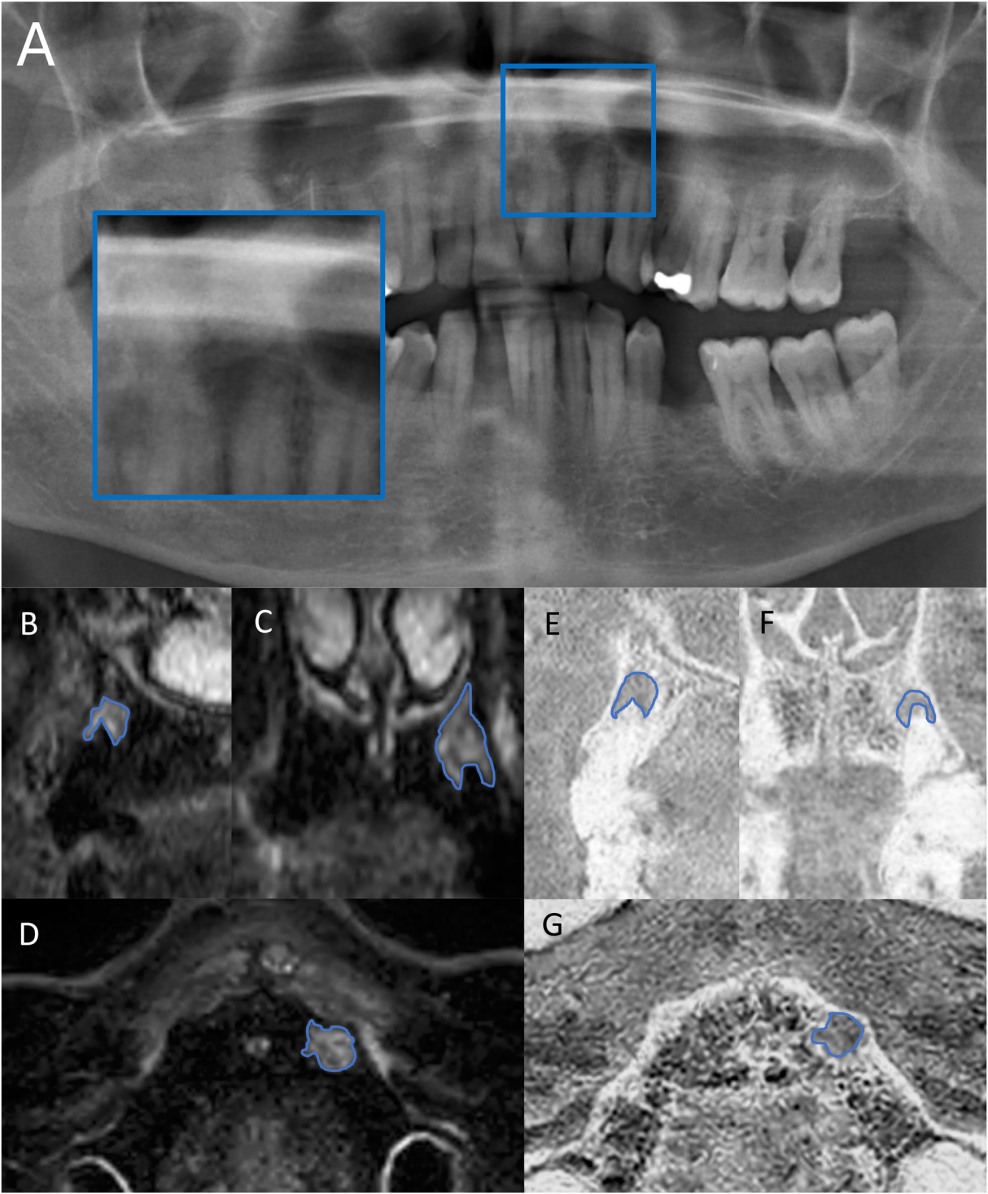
3D-segmentation of a periapical bone marrow edema of tooth 22 in a 64-year-old man. A periapical radiolucency is present in OPT (A) and was rated as PAI 5. Sagittal (B), coronal (C) and axial (D) reconstructions of a 3D STIR sequence of the same tooth showing a bright periapical edema at tooth 22 with a volume of 200 mm^3^. Sagittal (E), coronal (F) and axial (G) reconstructions of a 3D T1 sequence showing a periapical bone defect with a volume of 146 mm^3^ at the root of tooth 22
